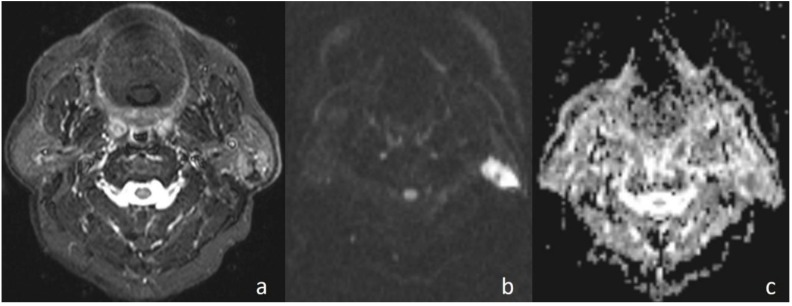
Warthin tumor. (a) Axial T2WI showing the mass localised in the superficial lobe and spreading into the deep lobe of the left parotid gland; (b) the lesion shows high signal intensity on DWI; and (c) the lesion is hypointense on the ADC map (ADC value of 0.88 × 10^−3^ mm^2^/s) and the tumor was falsely diagnosed as a malignant lesion.
